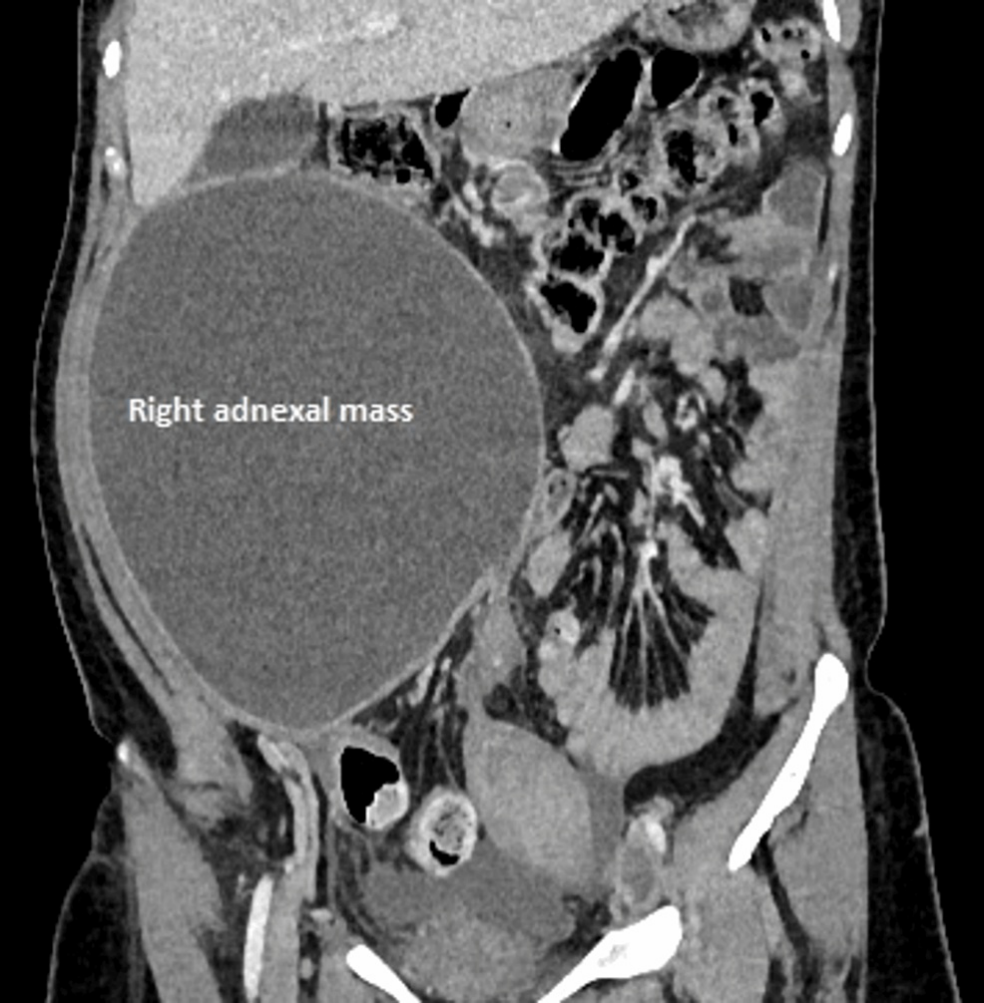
Contrast-enhanced computed tomography image Contrast-enhanced computed tomography of the abdomen and pelvis (coronal section) showing a right adnexal mass
